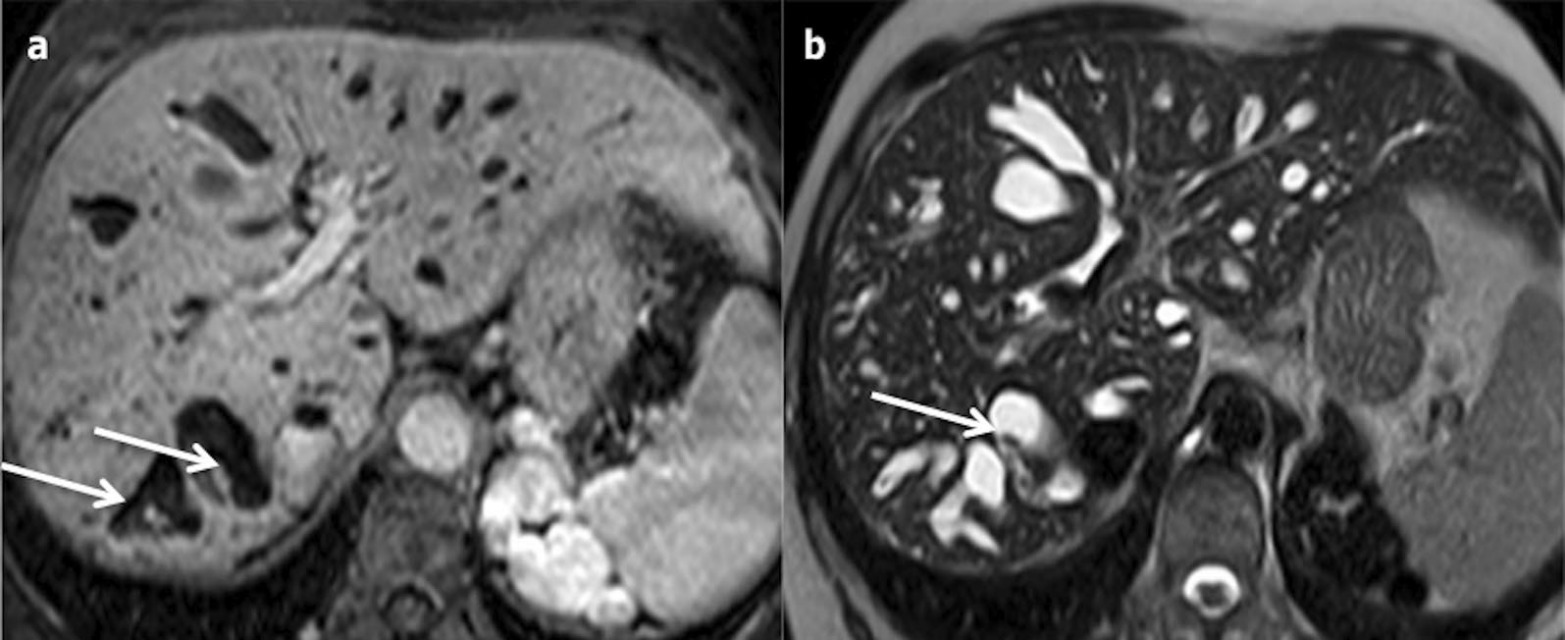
Caroli syndrome in a 53-year-old female. a Axial magnetic resonance imaging on portal venous phase shows peripheric fusiform dilated bile duct, hyperintense on axial T2-weighted magnetic resonance imaging (b), with the specific “central dot sign” (arrows). Note the presence of spleno-renal shunts due to portal hypertension
